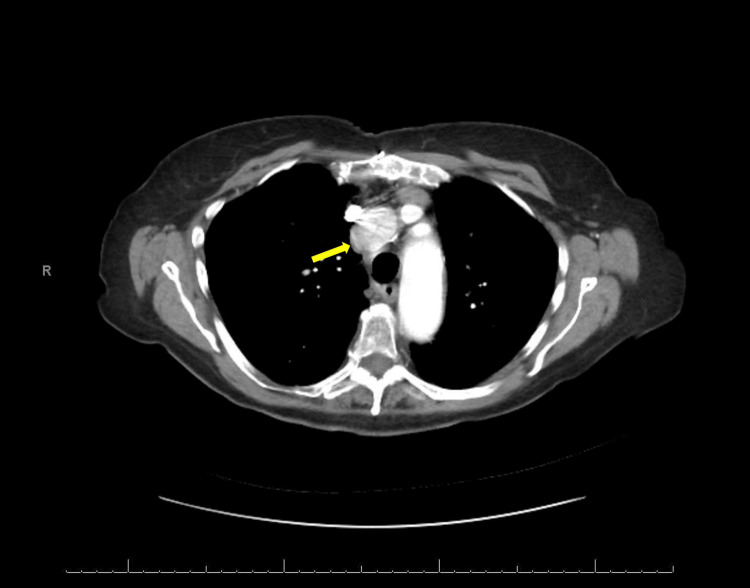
Axial cuts of a post-operative CT scan of the chest with IV contrast demonstrating a stable mass in the mediastinum (yellow arrow)
